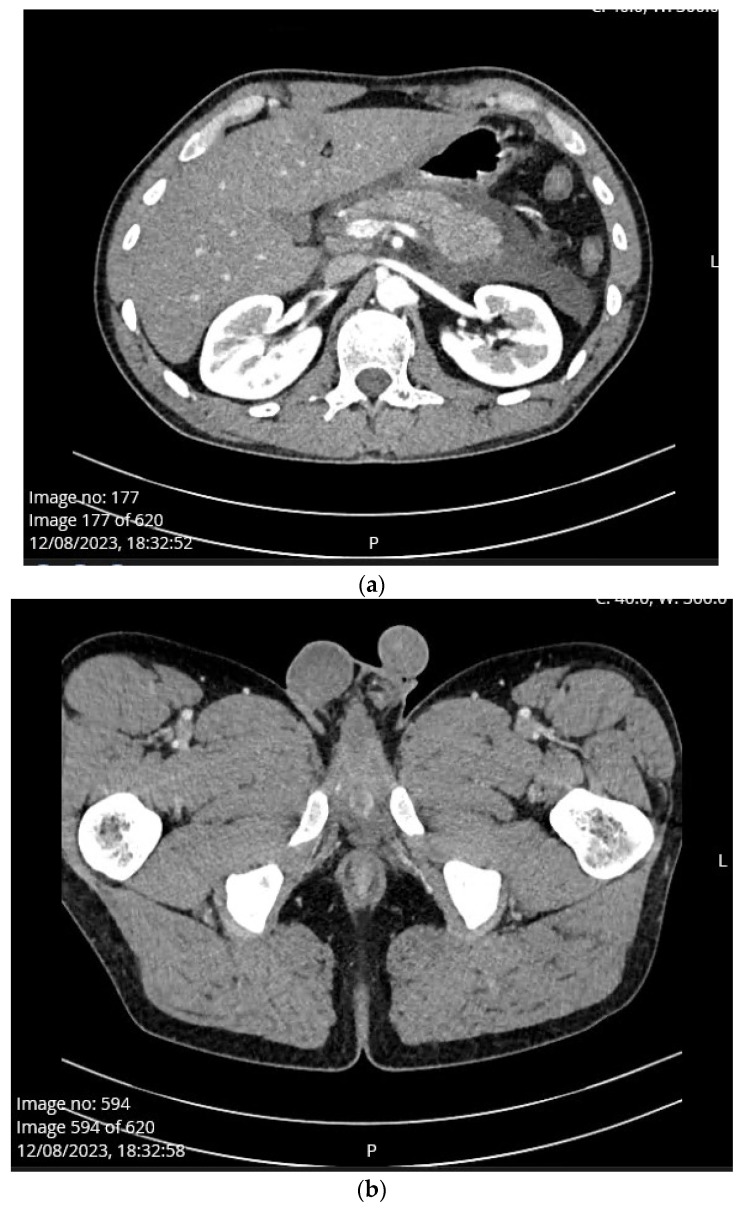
(a). Axial contrast abdominal CT scan showed fatty liver disease, pancreatic swelling, and acute peripancreatic fluid collections (interstitial edematous pancreatitis), fluid between the intestinal coils and along the anterior renal fascia, and minimal fluid in the Douglas space on the first day of admission. (b). Axial contrast CT scan showed there was no sign of penoscrotal swelling on the first day of admission.
